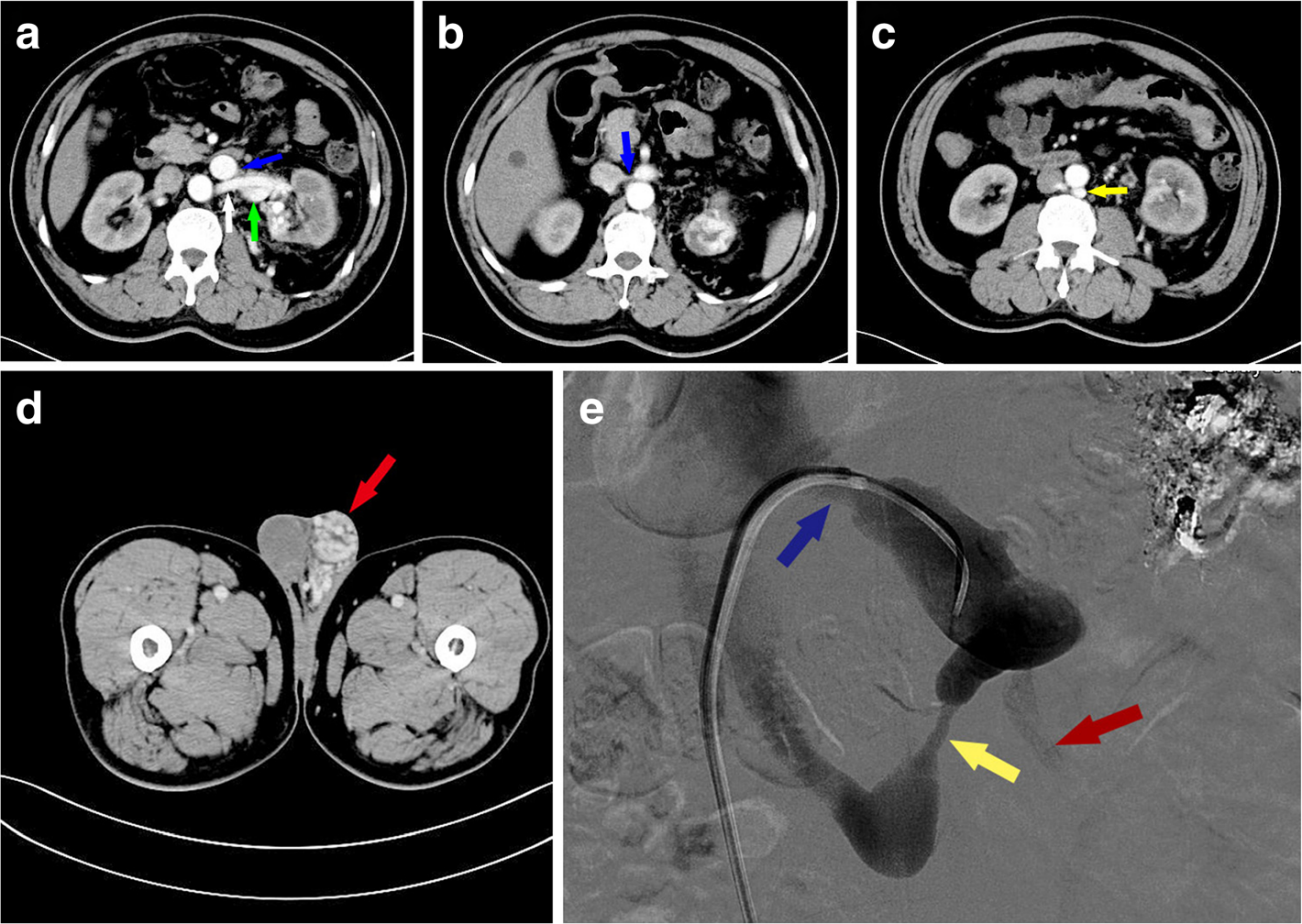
Additional radiological examination of the patient. a Enhanced computed tomography showed renal artery (*white arrow*), tortuous dilated vessel in left renal hilum (*green arrow*), and early enhanced renal vein (*blue arrow*). b Enhanced computed tomography showed early enhanced left renal vein with ostial stenosis (*blue arrow*). c Enhanced computed tomography showed dilated lumbar vein (*yellow arrow*). d Enhanced computed tomography showed enhanced left varicocele (*red arrow*). e Digital subtraction angiography showed lumbar vein (*yellow arrow*), light contrast in the left gonadal vein (*red arrow*) after selective embolization of the segmental renal artery, and dilation of the left renal vein ostia (*blue arrow*)
